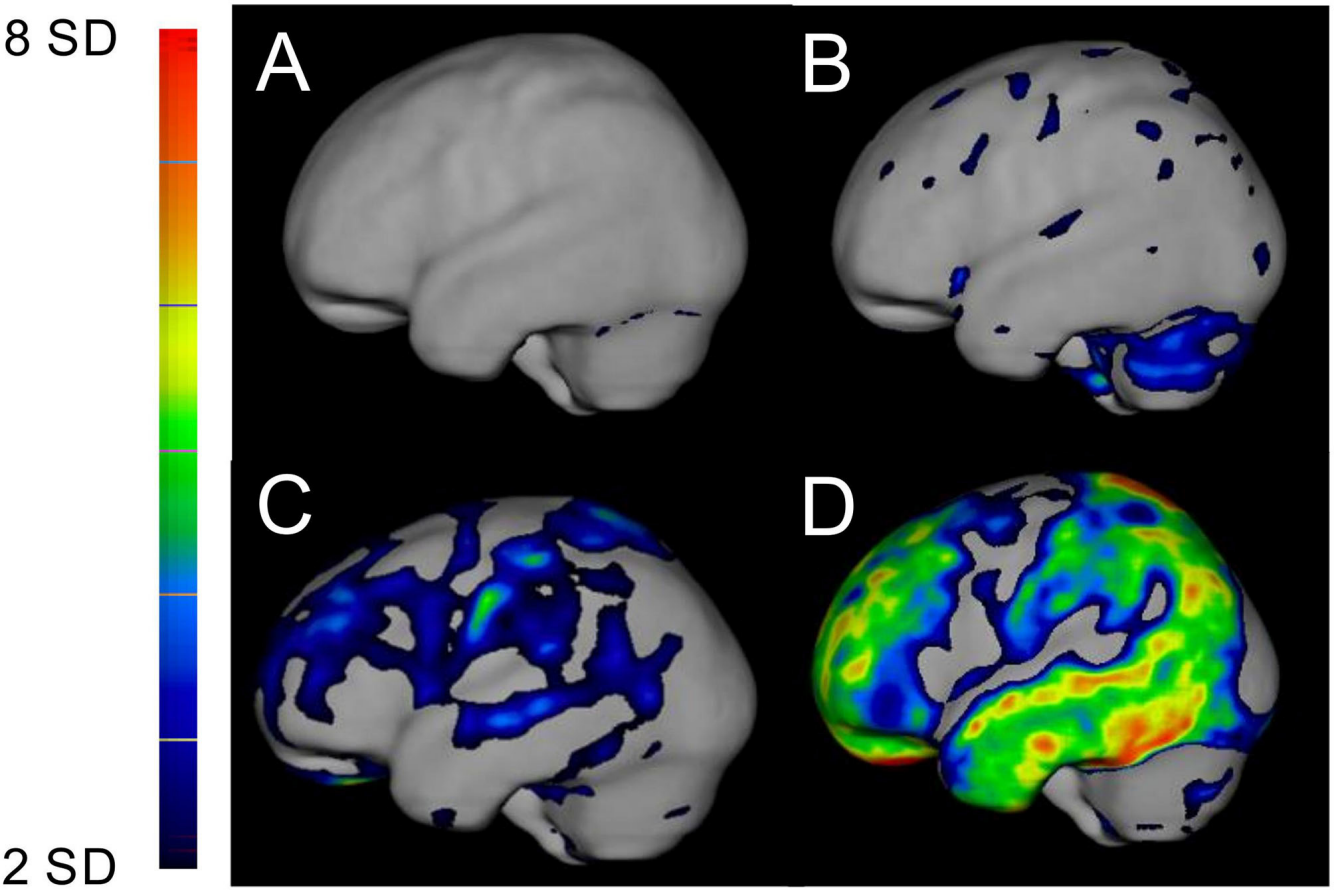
PET/CT examination of [^18^F]flutemetamol shown as Z-score in the brain in relation to pons from a left lateral view of the brain. The intensity of the uptakes is visualized on the left panel and is graded from low increased uptake in blue (Z-score +2SD) to high increased uptake in red (Z-score +8SD). (A) An ATTR V30M patient with 15 years of disease showing no increased uptake; (B) an ATTR V30M patient with 16 years of disease showing increased uptake in the cerebellum, (C) an ATTR V30M patient with 27 years of disease showing diffuse increased uptake in the brain, and (D) an Alzheimer's patient with generalized increased uptake in the frontoparietotemporal regions.
